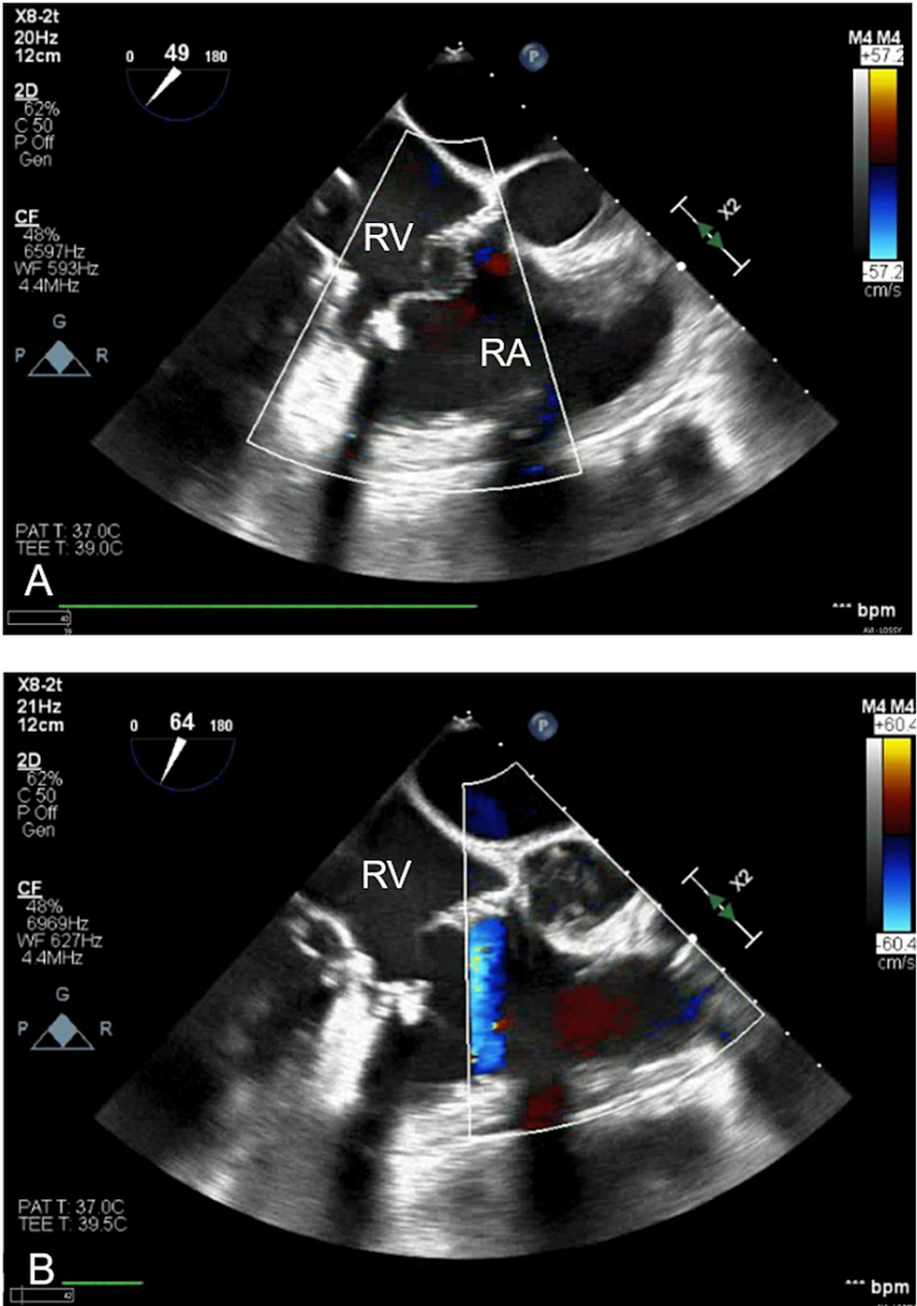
(A) Intraoperative TEE with color Doppler showing the bioprosthetic tricuspid valve. (B) Intraoperative TEE with color Doppler showing the bioprosthetic pulmonic valve. RV: right ventricle; RA: right atrium.
